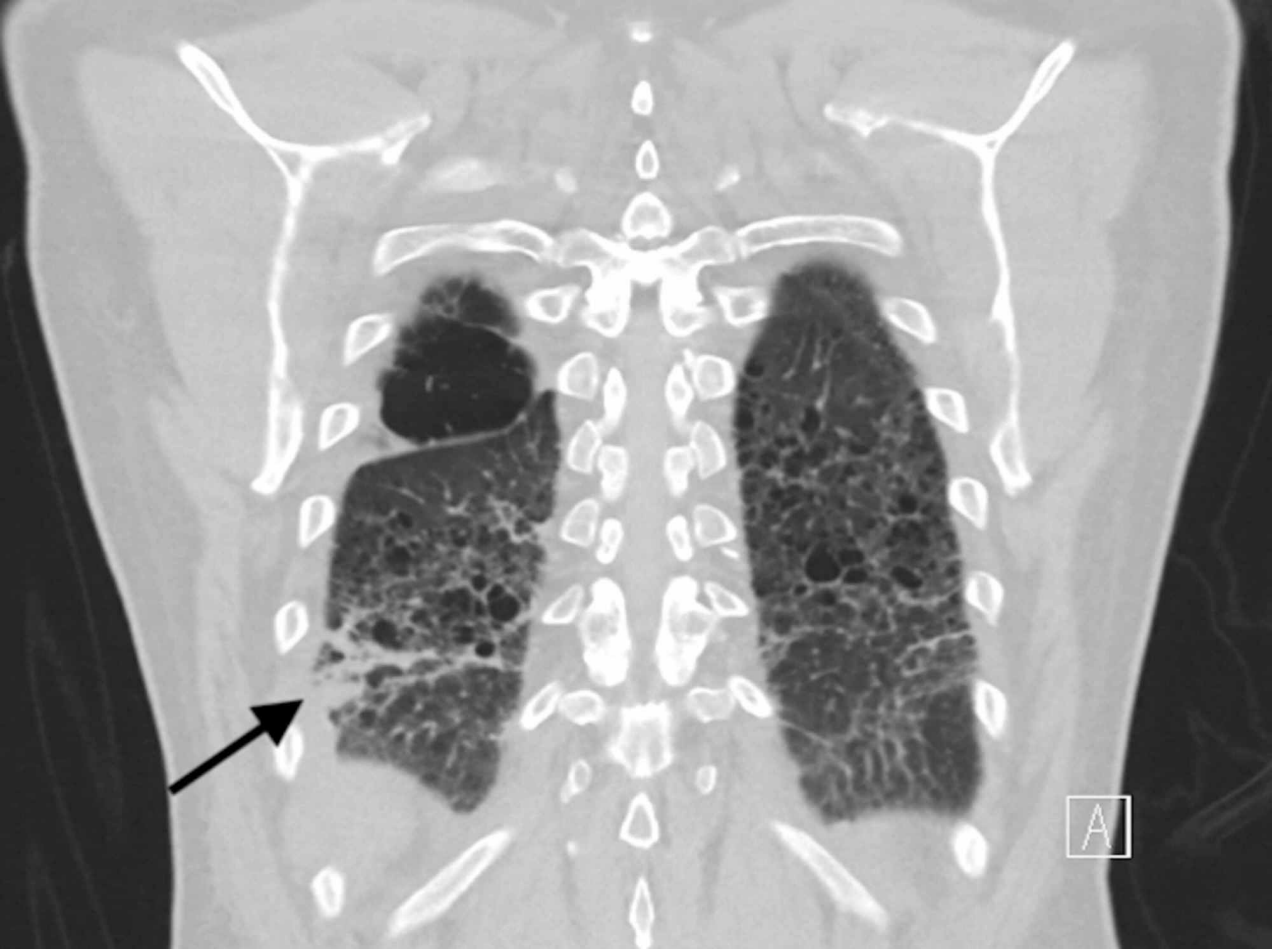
Emphysema and right lower lobe infiltrates on chest CT.
